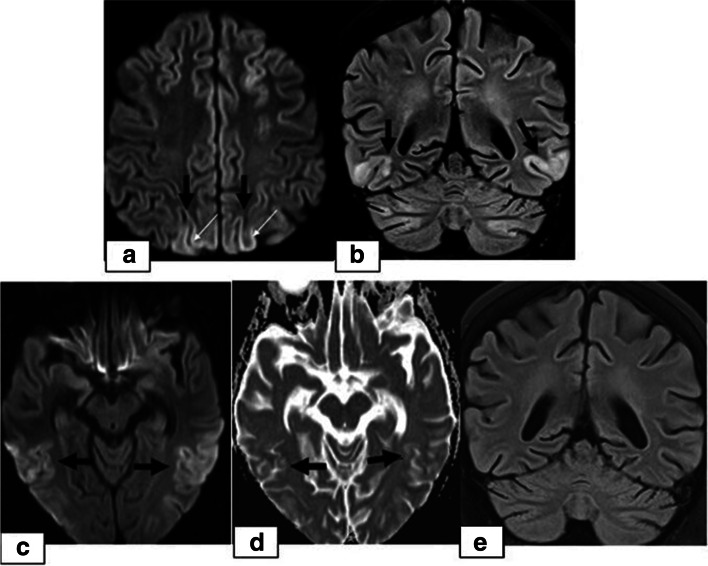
An 11-year-old boy presenting with seizures and visual disturbances 3 months after undergoing allogeneic HSCT for acute myeloid leukaemia. a Axial and b) coronal FLAIR images demonstrating bilateral symmetrical parietal (a) and occipital (b) cortico-subcortical hyperintensity (black arrows). Note the prominent involvement of cortical grey matter (1a, white arrow). c DWI and d) an ADC map showing a high signal (arrows) representing the T2 shine-through effect but not true restricted diffusion. e Follow-up coronal FLAIR image demonstrating complete resolution of vasogenic oedema in the occipital lobes after 3 months
